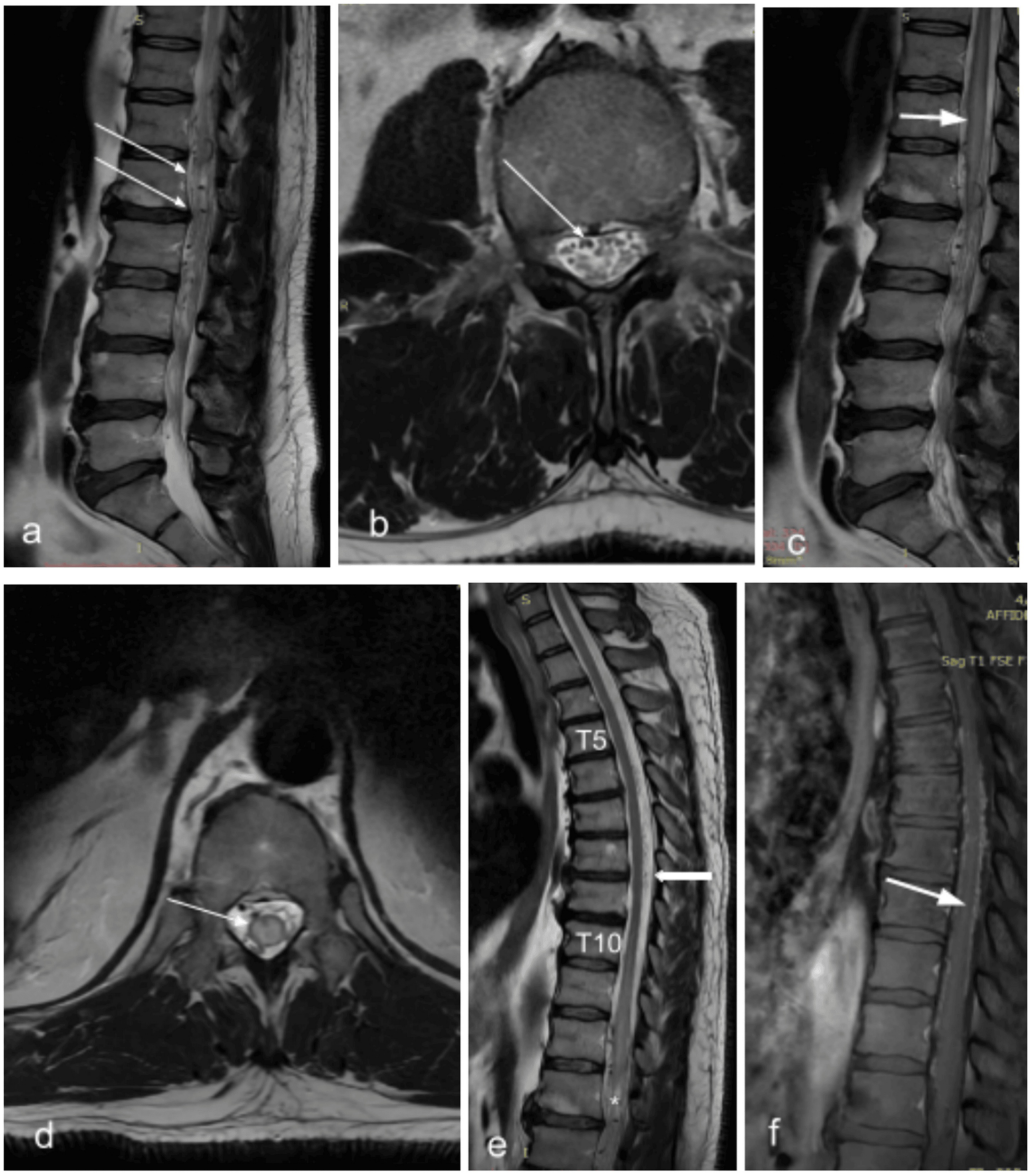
Pre-operative MRI of patient #1. a: Serpentinous intradural extramedullary flow voids in sagittal lumbar T2-weighted image (T2WI) (arrows). b: Descending vein between the rootlets of equina in axial T2WI (arrow). c: Sagittal T2WI from Th10 to L2 level, shows the intramedullary cord oedema (arrow). d: Same in the axial T2WI (arrow). e: Dorsal perimedullary venous plexus ascending as rostrally as levels Th5 to Th10 (arrow). f: post-gadolinium (PG) image discloses the intramedullary enhancement at the same levels as the oedema (arrow).
